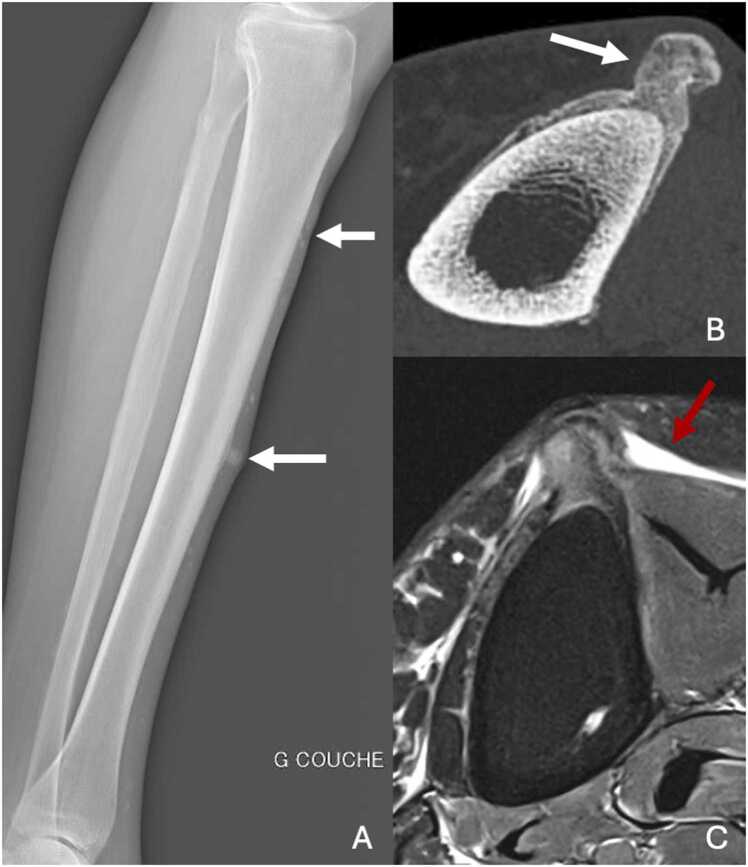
Voriconazole-induced periostitis in a 44-year-old woman with history of chronic end-stage renal disease treated by renal transplantation and invasive aspergillosis. A. Profile radiography of the left leg showing some plurifocal nodular calcifications (white arrows). B. CT image in the axial plane showing pronounced periosteal reaction with nodular aspect at the anterior edge of tibia (white arrow). C. Proton density-weighted fat-suppressed MR image in the axial plane showing hypersignal of periosteal reaction associated with adjacent neobursitis (red arrow).
